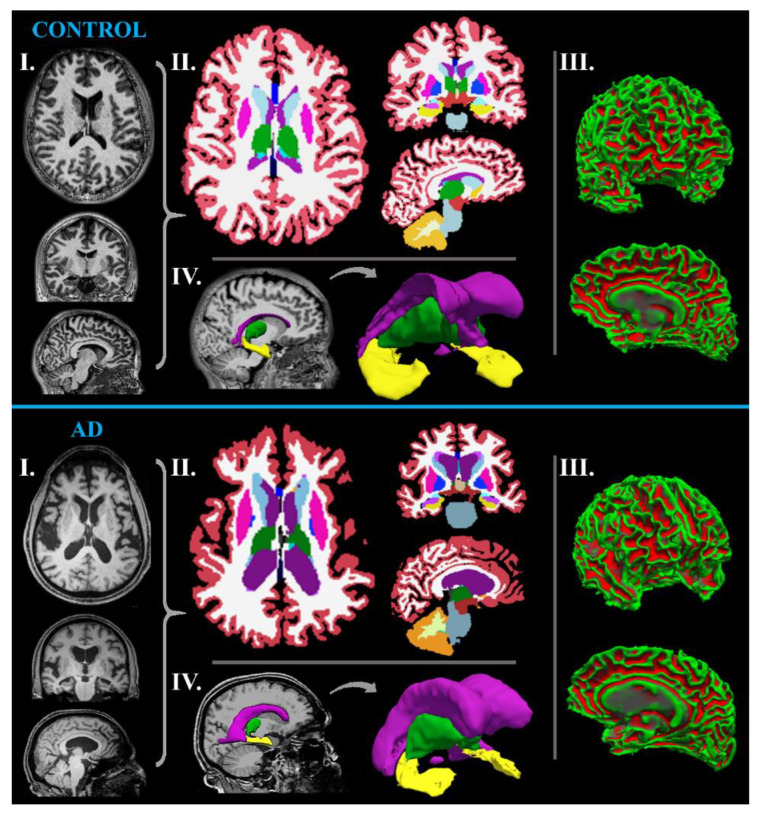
Example of MRI (I.; T_1_-weighted MRI at 3 Tesla MR-scanner) and MR-volumetric (II.; MR-volumetry performed on Freesurfer software) neuroimaging of a control subject (40-year-old female) and AD patient (42-year-old female) in axial, coronal, and sagittal sections. In AD, the apparent atrophy is shown across the white matter (III. 3D model of the whole brain) as well as in several brain structures (IV. 3D model of selected brain areas; atrophy of the hippocampus/yellow, atrophy of the thalamus/green, enlarged lateral ventricles/purple).
